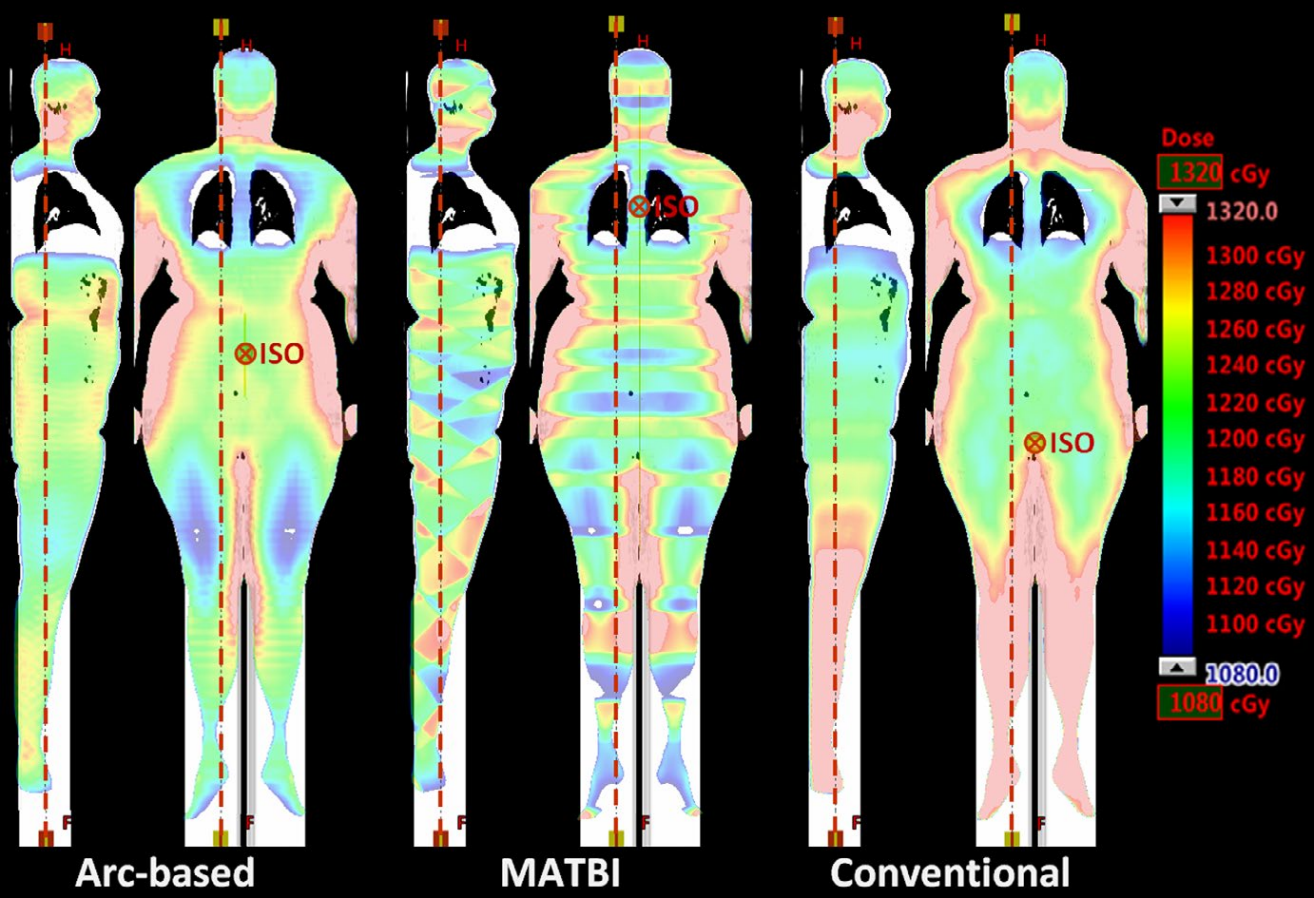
Calculated dose distribution for arc‐based, MATBI, and conventional extended source‐to‐surface distance techniques. Red dashed lines indicate the position of the corresponding coronal and sagittal planes. Isocenter position, which is different for each technique, is shown in every coronal plane. Rectangular regions in white in the inferior part of the images are due to the artificial expansion of the CT images necessary to have the full body in the same study
